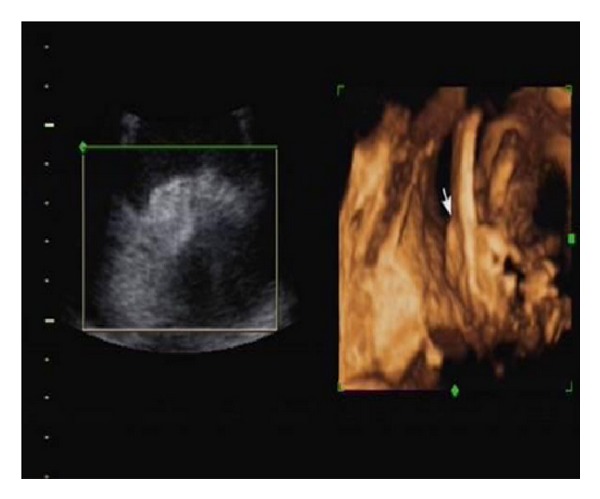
Three-dimensional sonogram of bilateral microtia.
